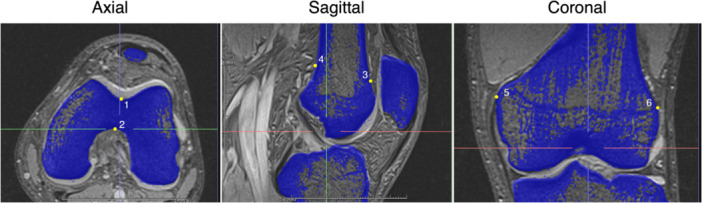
CT bone image (blue shadow) on MRI (black and white). The gaps between the CT and MR images were measured on the six bony borders: apex of the intercondylar notch (1) and bottom of the trochlea (2) on the axial slices; anterior (3) and posterior borders (4) of the femur on the sagittal slices; medial (5) and lateral borders (6) of the femur on the coronal slices. The mean gap was 0.44 ± 0.23 mm. CT, computed tomography; MRI, magnetic resonance imaging.
